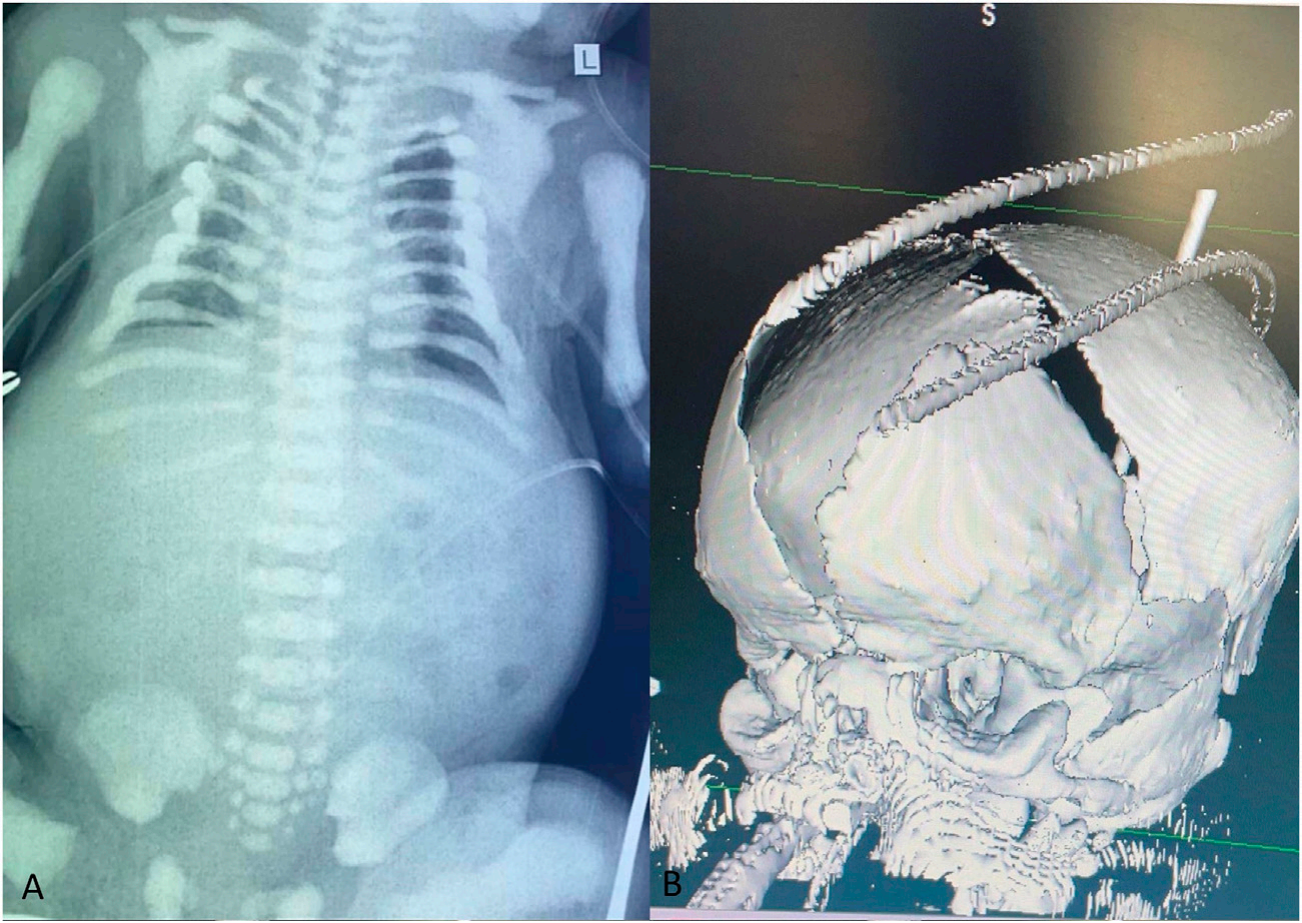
The chest X-ray in anteroposterior view indicates increased bone density in the ribs, vertebrae, scapulae, clavicles, humeri, and pelvis, which suggests the presence of generalized osteosclerosis (A). The brain three-dimensional CT scan reveals a widely open anterior fontanelle (B).
